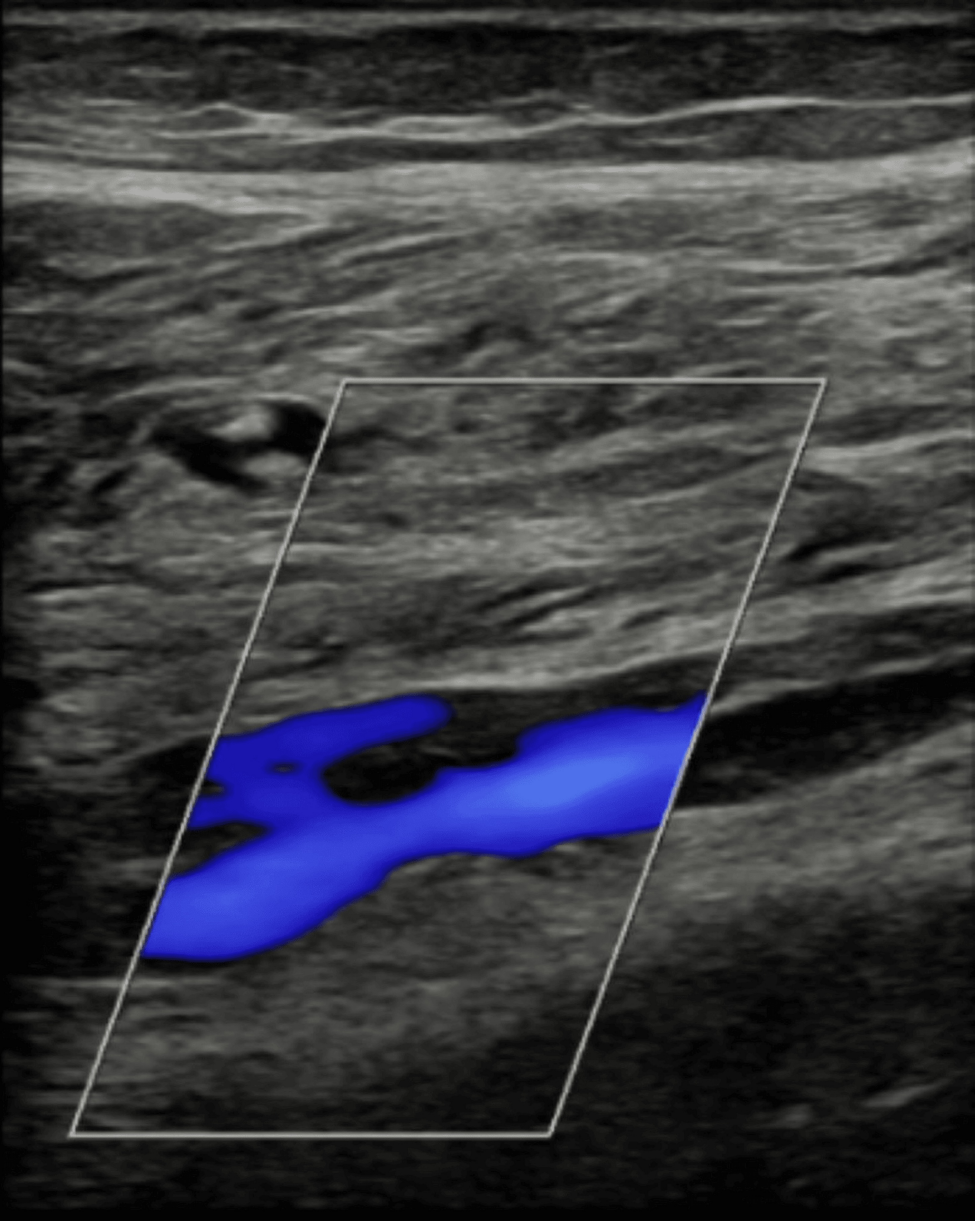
Shows a USS image of the right distal peroneal vein where a right-sided non-occlusive DVT was visualized. USS: ultrasonography scan; DVT: deep venous thrombosis
